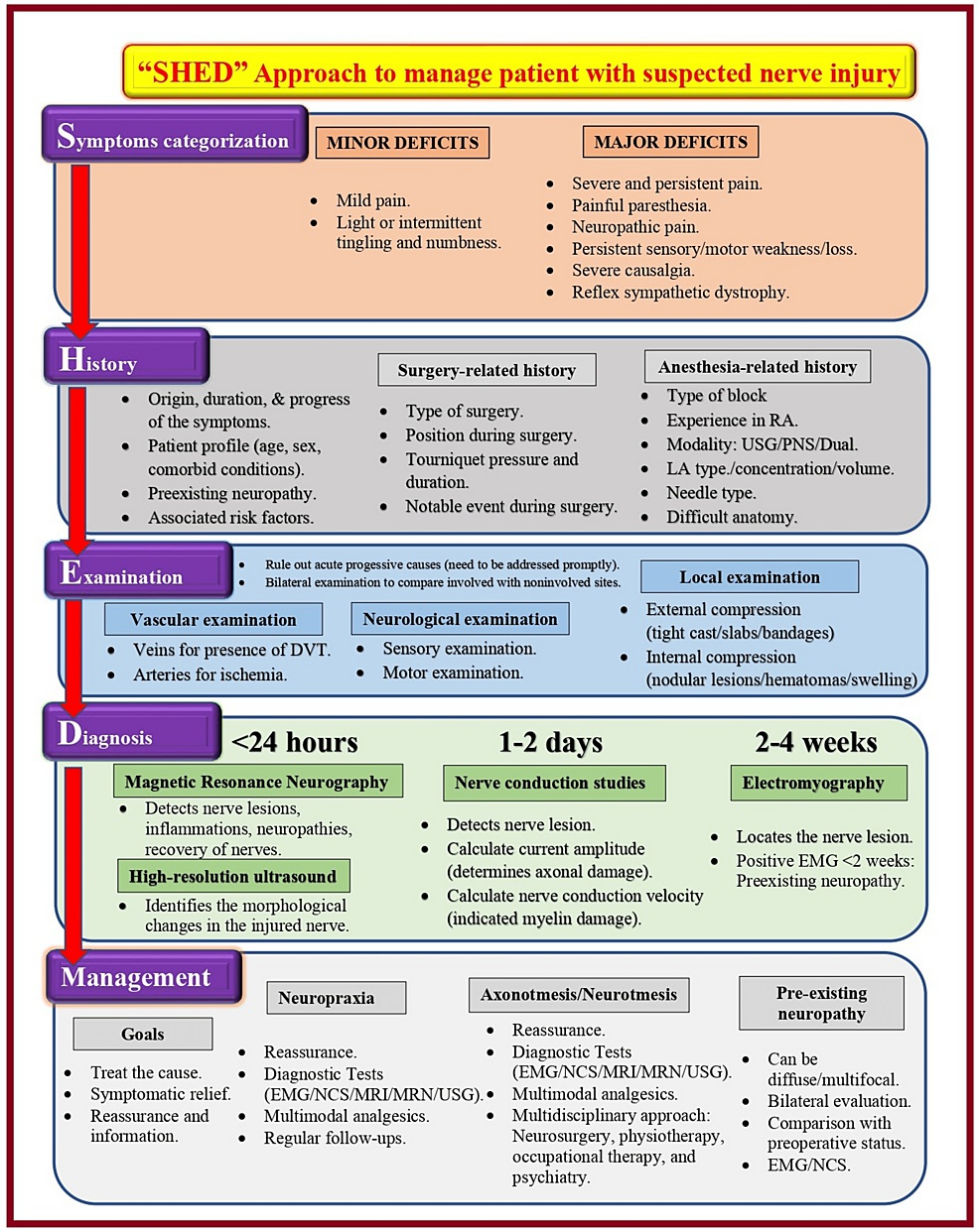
“SHED” approach to manage patients with nerve injuries RA: regional anesthesia; USG: ultrasound guidance; PNS: peripheral nerve stimulator; DVT: deep venous thrombosis; EMG: electromyography; NCS: nerve conduction; MRN: magnetic resonance neurography Source: This figure was created by the first author (KS).
